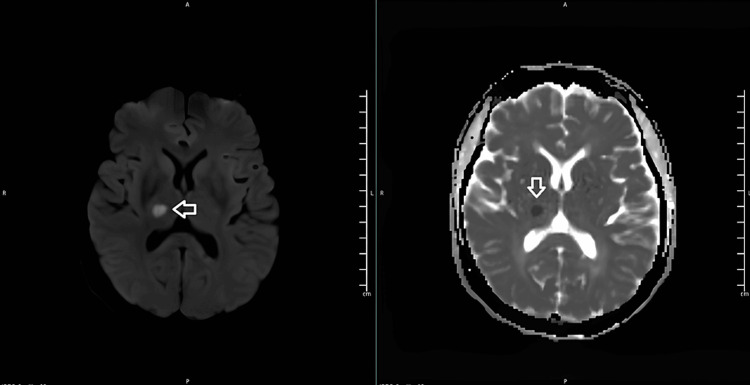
An MRI head DWI (left) and ADC (right) images showing a right thalamus acute to early subacute infarct. DWI - Diffusion-weighted imaging, ADC - apparent diffusion coefficient
